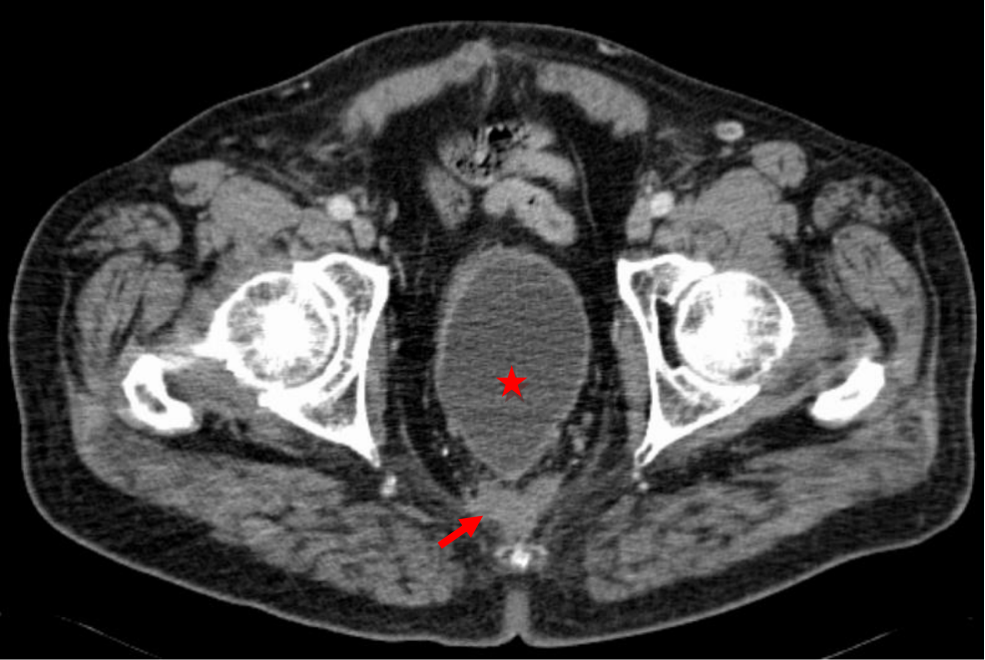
Pelvic CT scan of a patient who underwent abdominal perineal resection. Posteriorly to the bladder, there is an ill-defined irregular soft tissue mass corresponding to post-surgical fibrosis (*arrow*). Note the posterior displacement of the bladder (*star*) into the pre-sacral space
